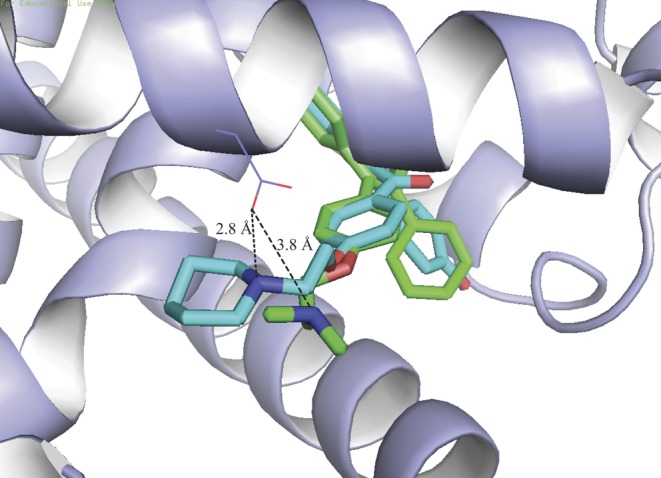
Role of Asp351 in the different activity of tamoxifen and raloxifene. Overlay of X-ray structures of 4-hydroxytamoxifen (green) and raloxifene (aqua) bound to ERα (data from Shiau *et al.* (1998) and Brzozowski *et al.* (1997), respectively). The distance from Asp351 to the dimethylamine in 4-hydroxytamoxifen (3.8 Å) is 1.0 Å longer than to the piperidine in raloxifene.
